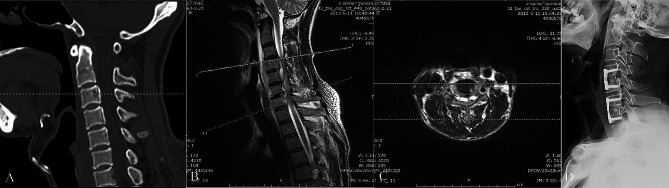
(A) Sagittal computed tomography image shows no sign of bone destruction. (B, C) Magnetic resonance imaging scans show the formation of paravertebral and epidural abscesses and spinal cord compression at the C4-7 levels. (D) Fifteen months after surgery, the C4-5 and C6-7 intervertebral bone grafts achieved complete fusion
